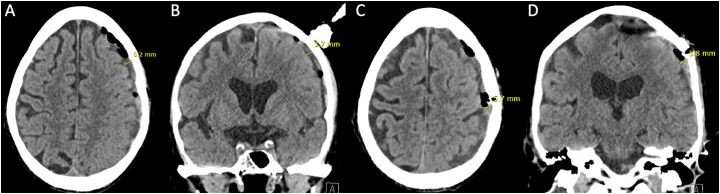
Head CT, after second SEPS. Caption: Head CT after implantation and removal of the second SEPS 2 cm posterior and 1 cm lateral to the first SEPS, with evacuation of a separate, lateral subacute SDH. Panel A and B show the more anterior component of the SDH and unchanged location of the first SEPS, with improvement of the convexity SDH to 4–6 mm. Panel C and D show the more posterior component of the SDH, which has also improved to 6 mm in thickness. CT = computed tomography; SEPS = subdural evacuation port system; SDH = subdural hematoma.
